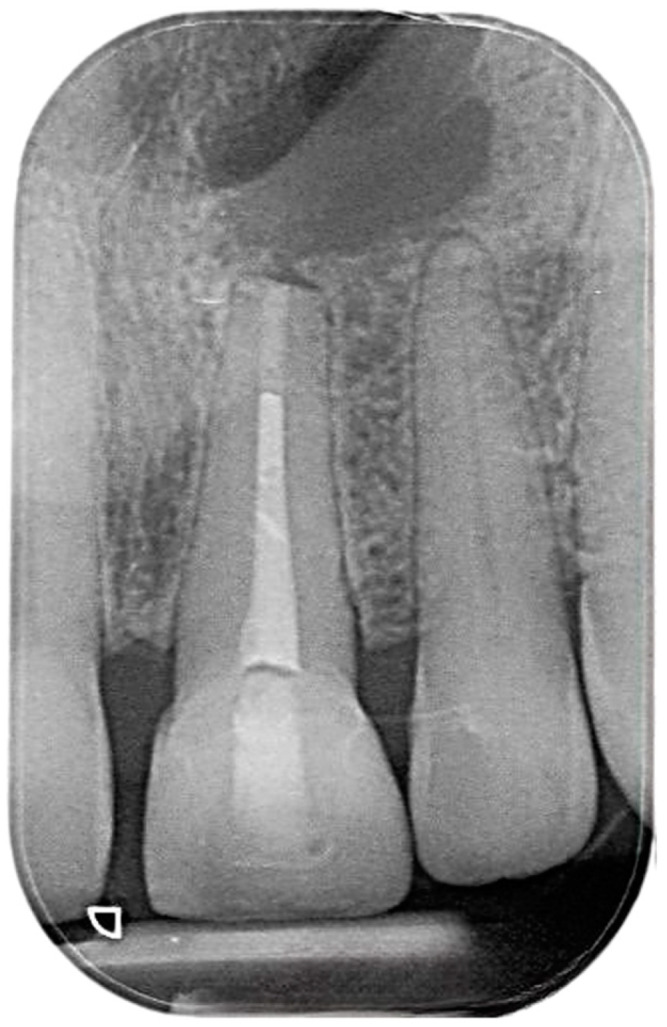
One-year-review, post-surgery intraoral periapical radiograph the upper left central and lateral incisors.
